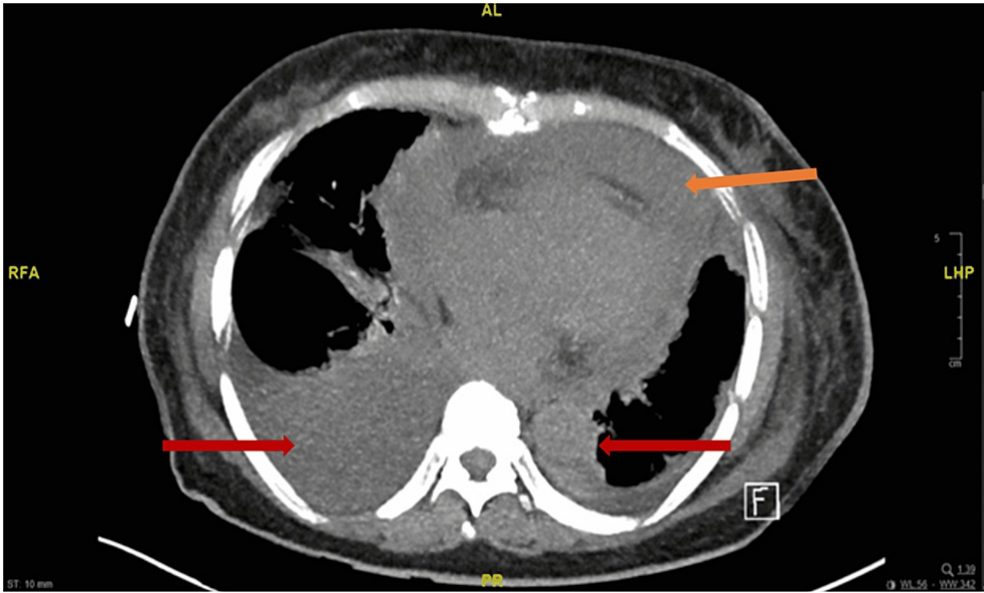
CT scan of the chest confirming the large pericardial effusion (orange arrow) and a large right and small left pleural effusion (red arrow).
